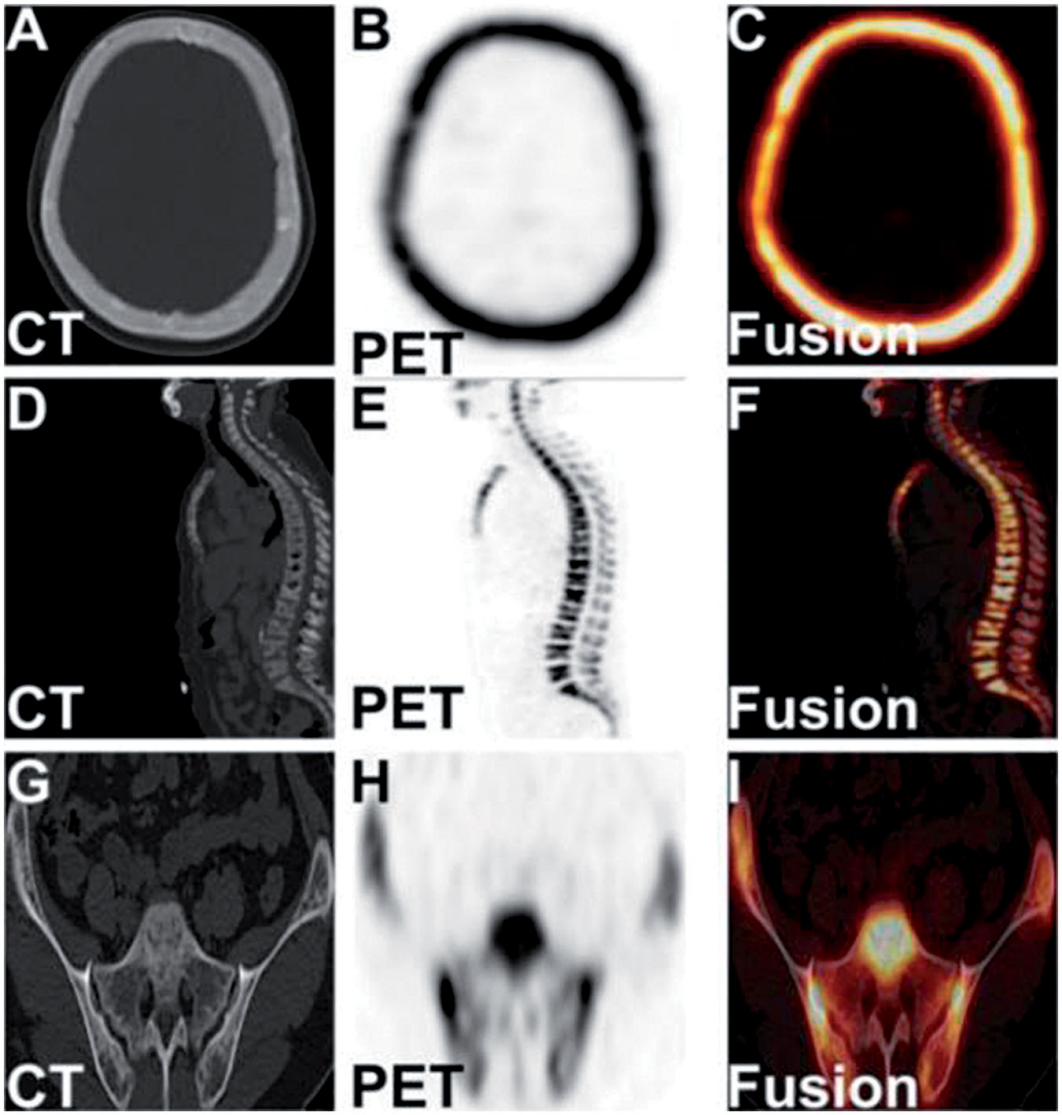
The tomography images of the skull, lumbar spine, and bilateral ilium in ^18^F-NaF PET/CT imaging.
